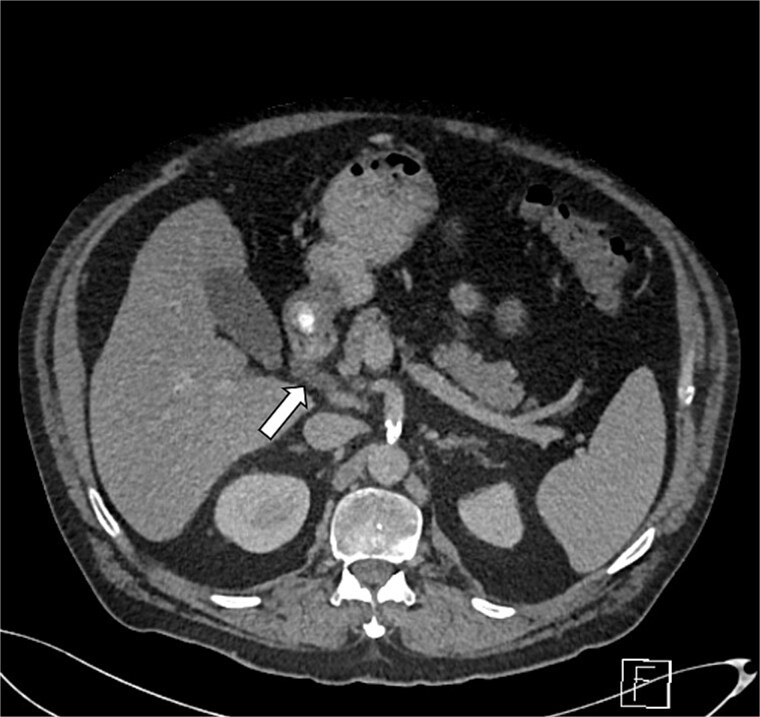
Axial section of abdominal CT scan. The axial section of the abdominal CT scan shows inflammatory fat stranding around the head and uncinate process of the pancreas.
